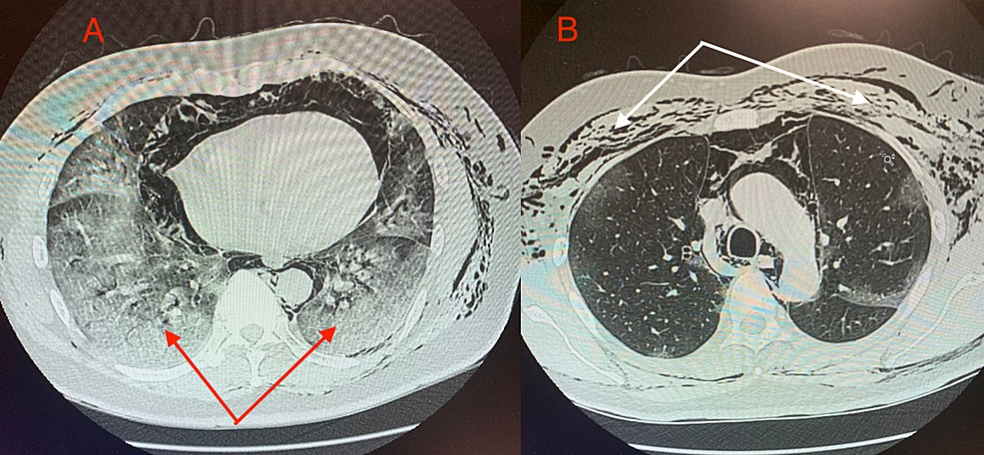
Coronal lung CT scan A) The image shows ground-glass air space opacities throughout bilateral lung bases consistent with COVID-19-positive pneumonia as shown by the red arrows. B) The image shows subcutaneous emphysema involving the right and left chest in a symmetric fashion as shown by the white arrows CT: computed tomography; COVID-19: coronavirus disease 2019
